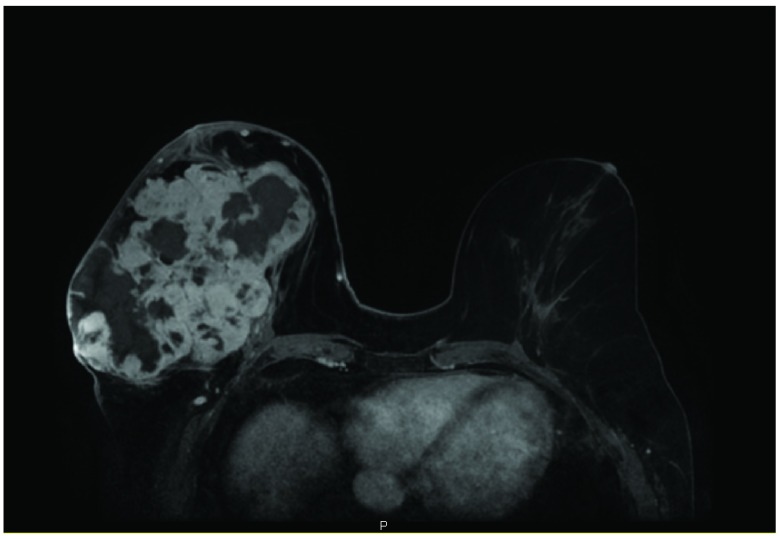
Breast MRI with contrast, axial view. In the right breast, there is a large lobulated heterogeneously enchancing mass with multiple areas of non-enchancement consistent with extensive necrosis. On axial image, the mass measures 15 cm × 9 cm × 13 cm. In the left breast, there is no suspicious mass. No suspicious adenopathy is seen in the axillae bilaterally.
